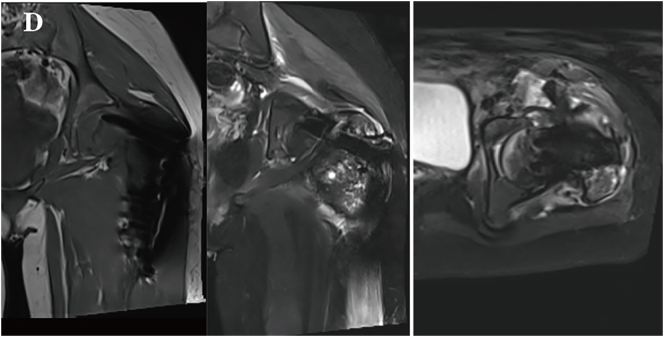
MRI showed cortical expansion with resorption and the presence of a soft tissue mass.
